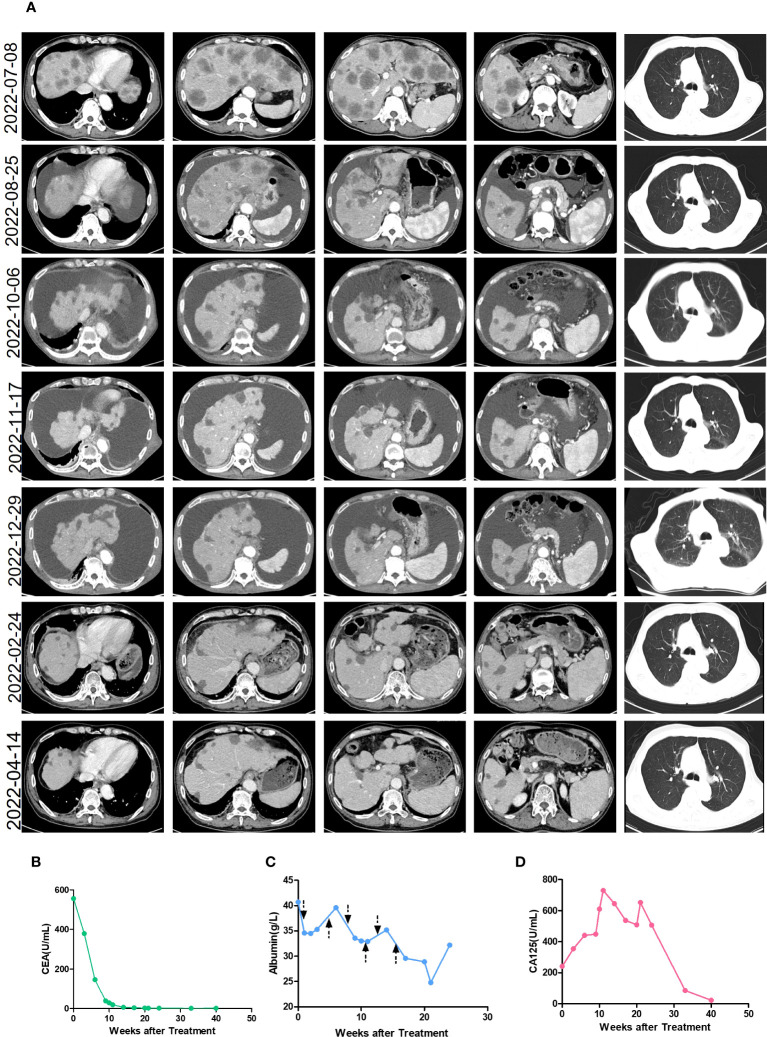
The clinical characteristics during treatment. (A) The enhanced CT images of the patient during treatment. The treatment began on 14 July 2022 and stopped on 29 November 2022. (B) The level of CEA during the treatment. The treatment began in week 0 and stopped in week 20. (C) The level of albumin during the treatment. The treatment began in week 0 and stopped in week 20. The arrow refers to the time point of the ASKB589 infusion. (D) The level of CA125 during the treatment. The treatment began in week 0 and stopped in week 20.
